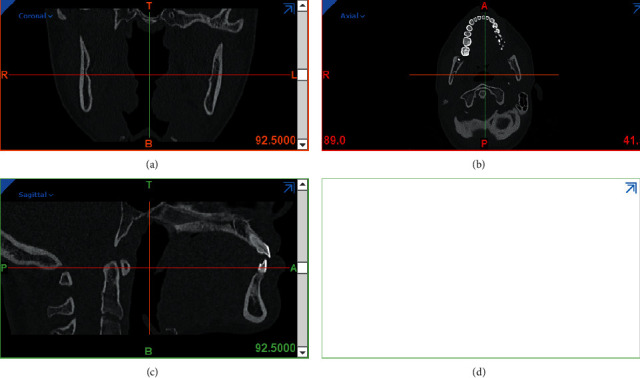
The CT scan used in this study.
